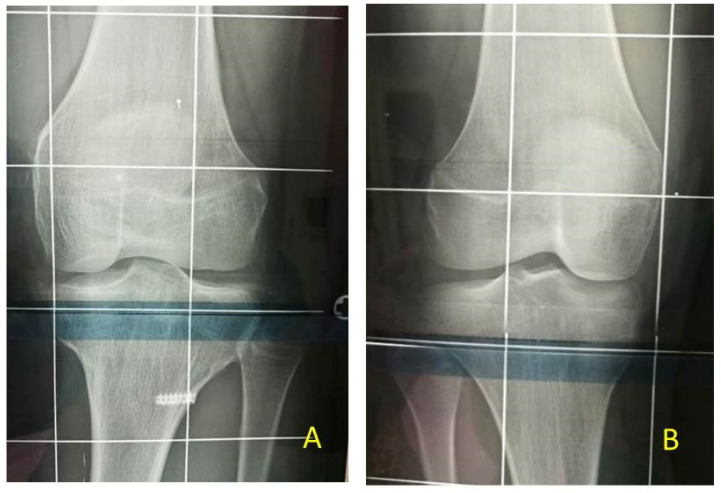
Same patient shown in Figure 10: (A) X-ray of left knee at follow-up after PTE (temporary proximal tibia epiphysiodesis) and DFE (temporary distal femur epiphysiodesis). No change in morphology of tibial plateau during growth; (B) X-ray of right knee for comparison.
